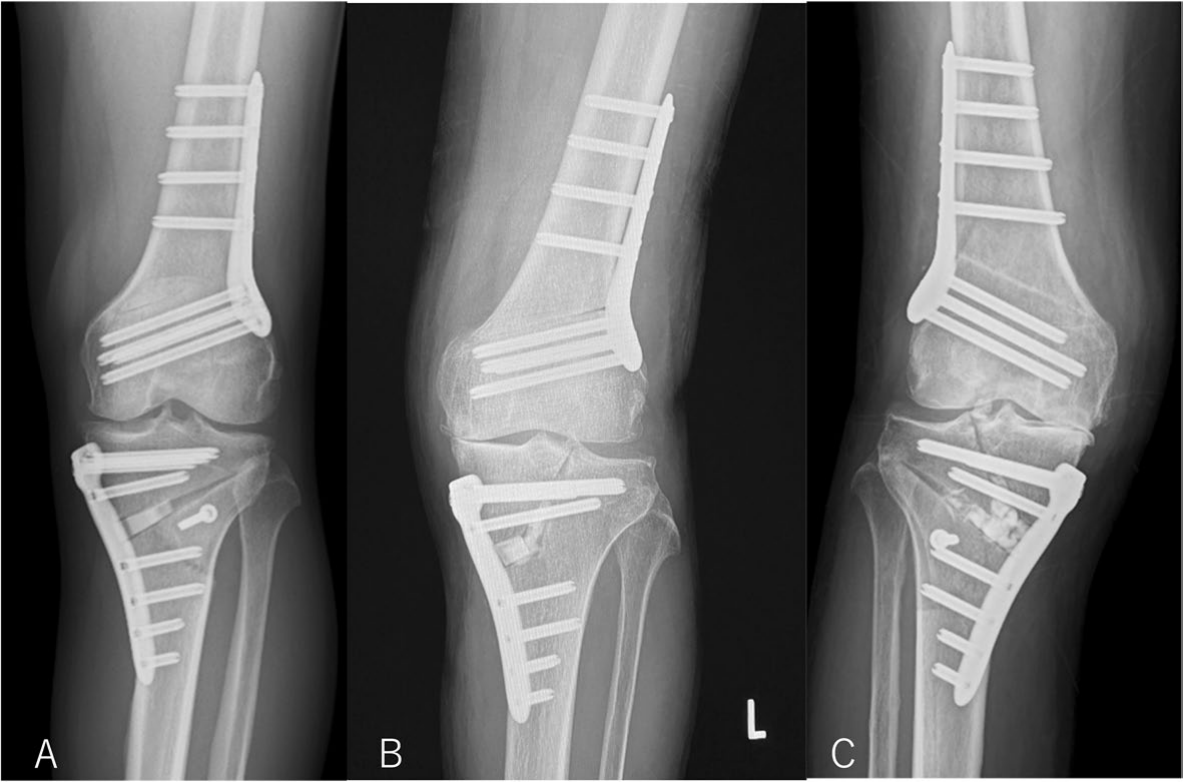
Postoperative weight-bearing anteroposterior radiographs after DLO, A: LCW-DFO + OWDTO (left knee), B: LCW-DFO + TCVO (left knee), C: LCW-DFO + TCVO + OWDTO (right knee), DLO: double level osteotomy, LCW-DFO: lateral closed-wedge distal femoral osteotomy, TCVO: tibial condylar valgus osteotomy, OWDTO: open wedge distal tuberosity osteotomy
